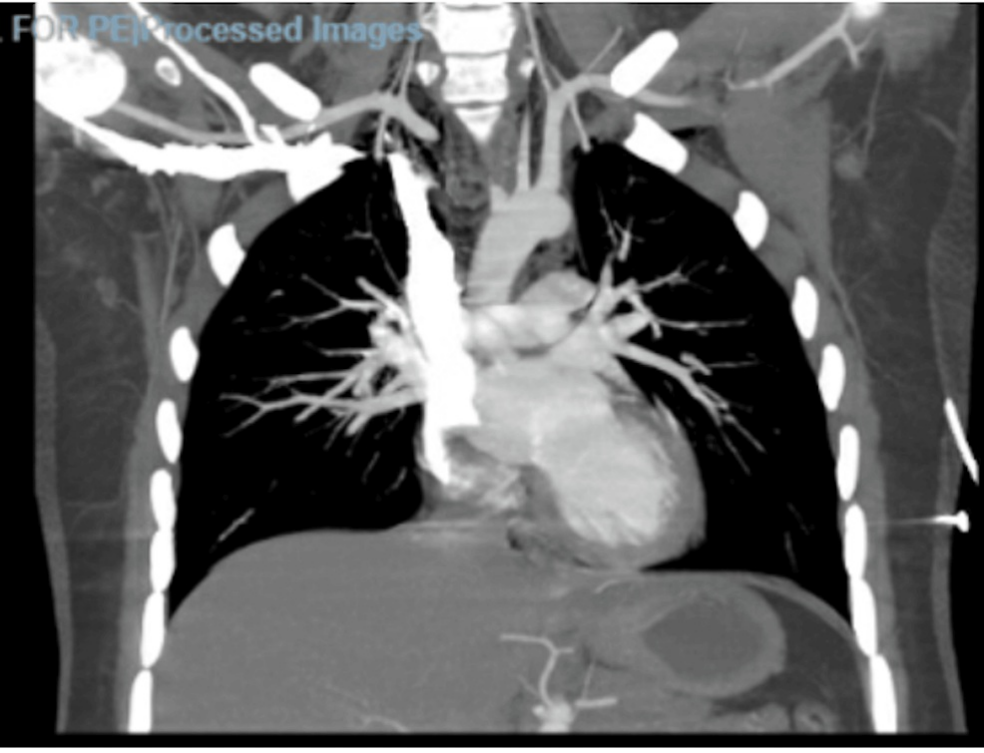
Findings of CT angiography of the chest (coronal view). There is diffuse subcutaneous emphysema in the neck and pneumomediastinum. There is diffuse esophageal wall thickening, and esophageal perforation cannot be excluded. There is no significant mediastinal or hilar adenopathy. No pulmonary emboli, pulmonary infiltrates, pleural effusion, or pneumothorax can be identified.
